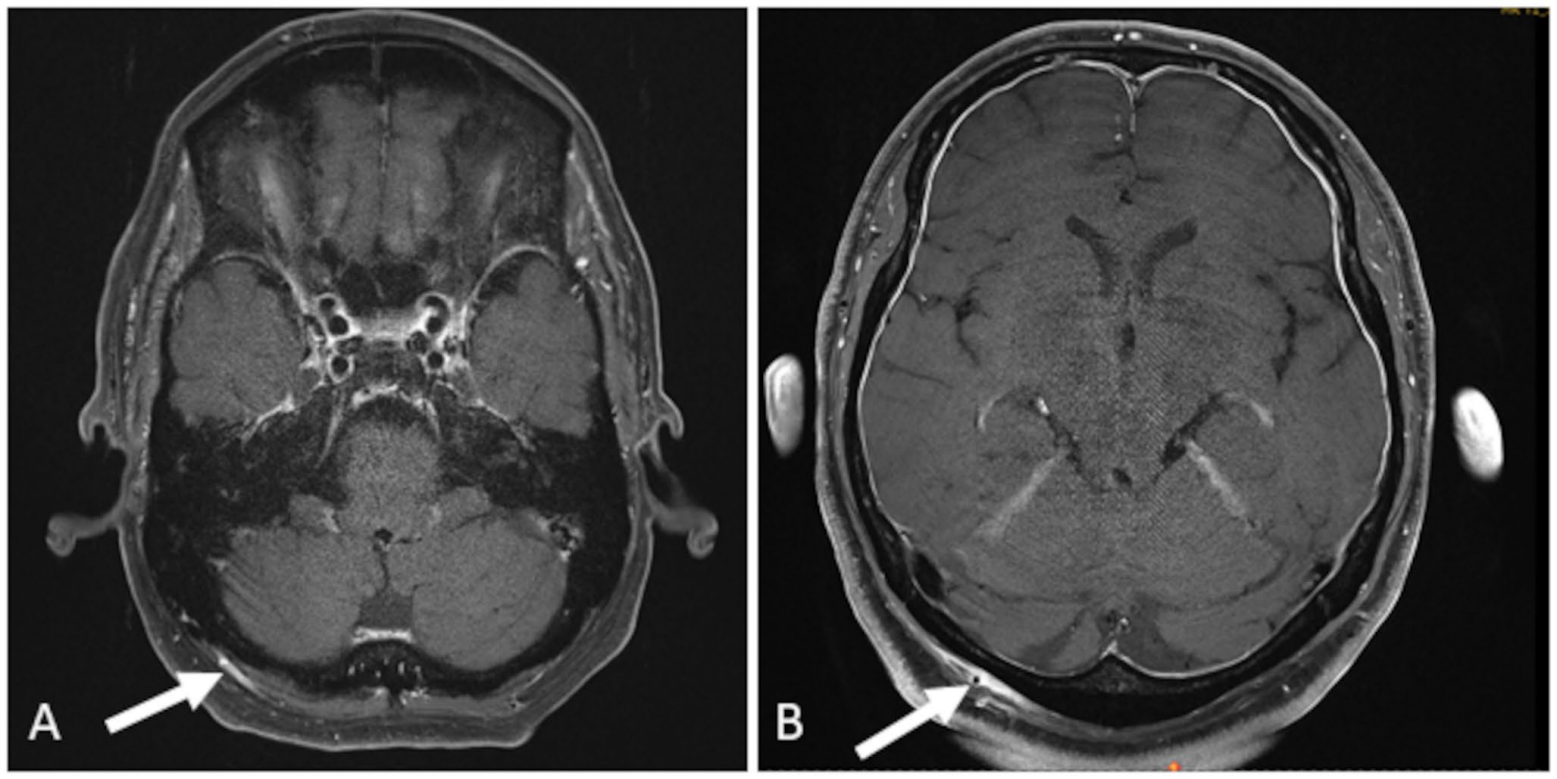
Isolated inflammatory affection of the occipital artery High resolution MRI shows vivid enhancement both of the vessel wall of the right occipital artery and of the surrounding perivascular soft tissue (A, B, arrow) in two GCA patients. The superficial temporal arteries are unremarkable in both patients
